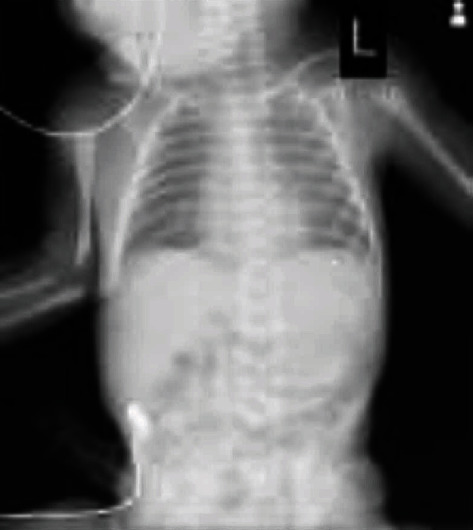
Chest radiograph of preterm infant with respiratory distress syndrome (RDS).
